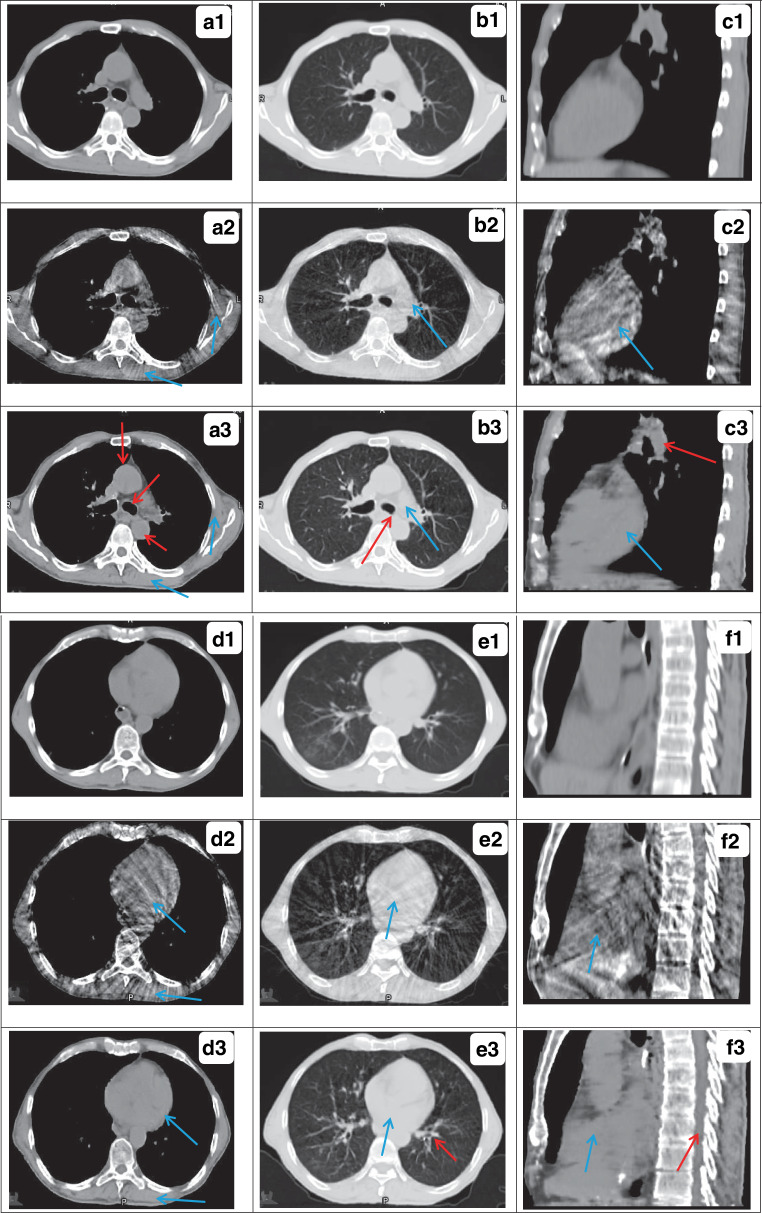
Quality comparison of deformed CT (dCT), cone-bean CT (CBCT), and synthetic CT (sCT) images generated by RegGAN for the same patient in axial or sagittal images. The image types are marked with numbers: *1* stands for dCT, *2* for CBCT and *3* for sCT. Respectively, in the upper right corner of the image, the same axial or sagittal planes are labeled as a, b, c, d, e, and f in turn. *Blue arrows* serious artifacts, *red arrows* good tissue continuity. The display window in second the column is [−1200 300] Hounsfield units (HU; lung window), and the display window in other rows is [−400 400] HU
